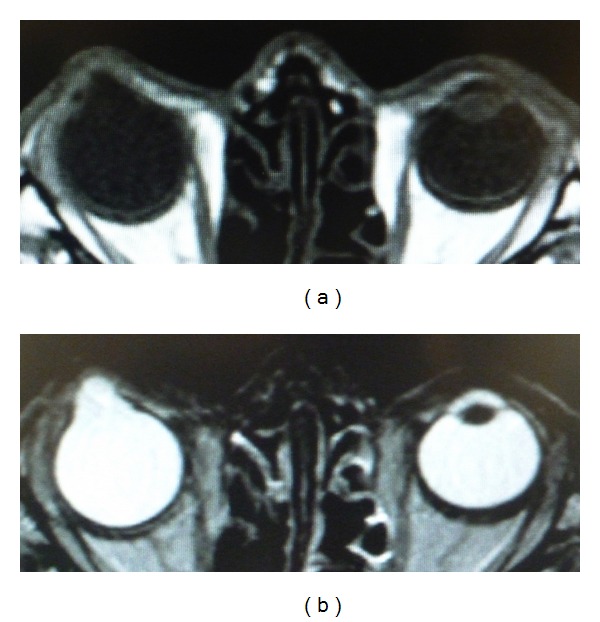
MRI image. The inside of the bulging lesion was not occupied with a tissue mass and lens was undetected. (a) T1, (b) T2.
